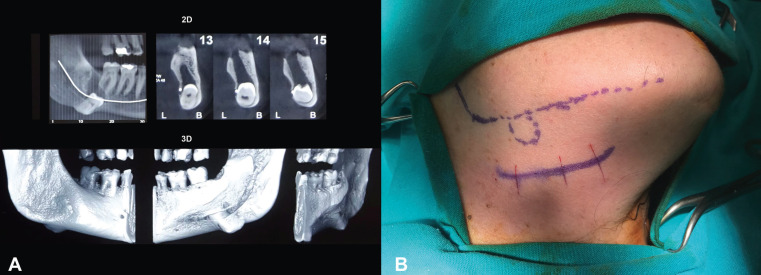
A) Selected 2D and 3D views from patient’s CBCT demonstrating that the impaction is mainly affecting the lingual plate of the lower border of the right mandibular body. B) Careful planning of the surgical procedure intraoperatively. The dotted straight line represents the lower border of the right mandible. The continuous straight line represents the skin incision (2cm below the lower border of the right mandible). The dotted circle represents the location of the impacted tooth.
